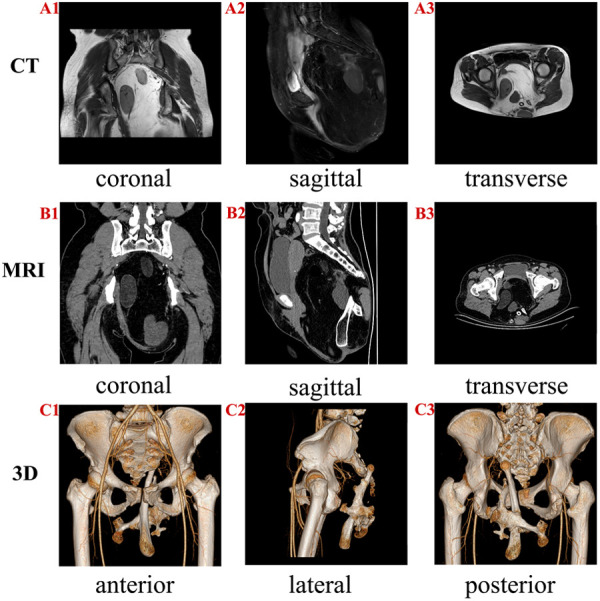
Magnetic resonance imaging (MRI) and 3D computed tomography (CT) of the patient. (A1–A3) CT showing different views of the teratoma. A huge space-occupying lesion exhibiting different densities can be observed in the pelvic cavity. A clear boundary is observed between the teratoma and pelvis. (B1–B3) MRI showing various views of the teratoma. The massive sacrococcygeal multiloculated lesion comprises a large amount of fat, sebaceous material, and long bone. (C1–C3) Various 3D views of the bone reconstruction based on CT; the 3D reconstruction allowed the visualization of the spatial positions of the bones within the teratoma as well as the lesion's relationship with the pelvis.
